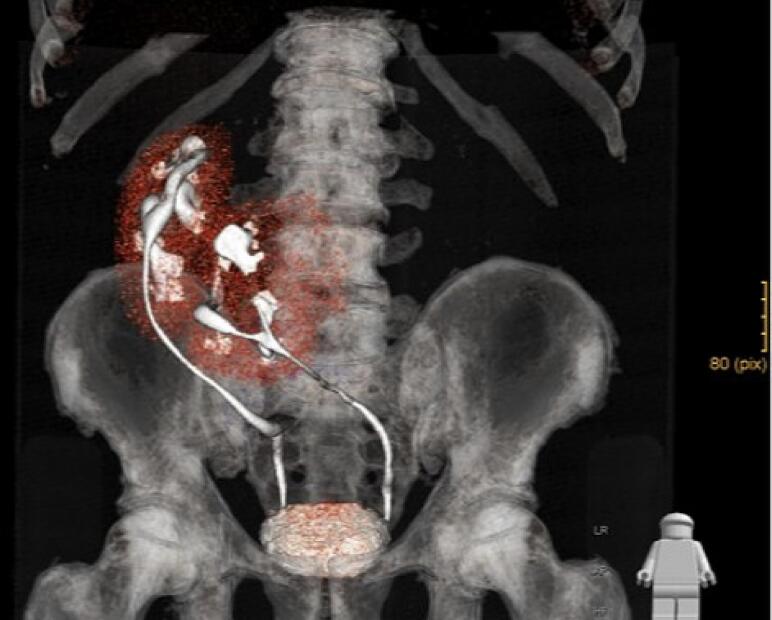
Preoperative CT urogram.
